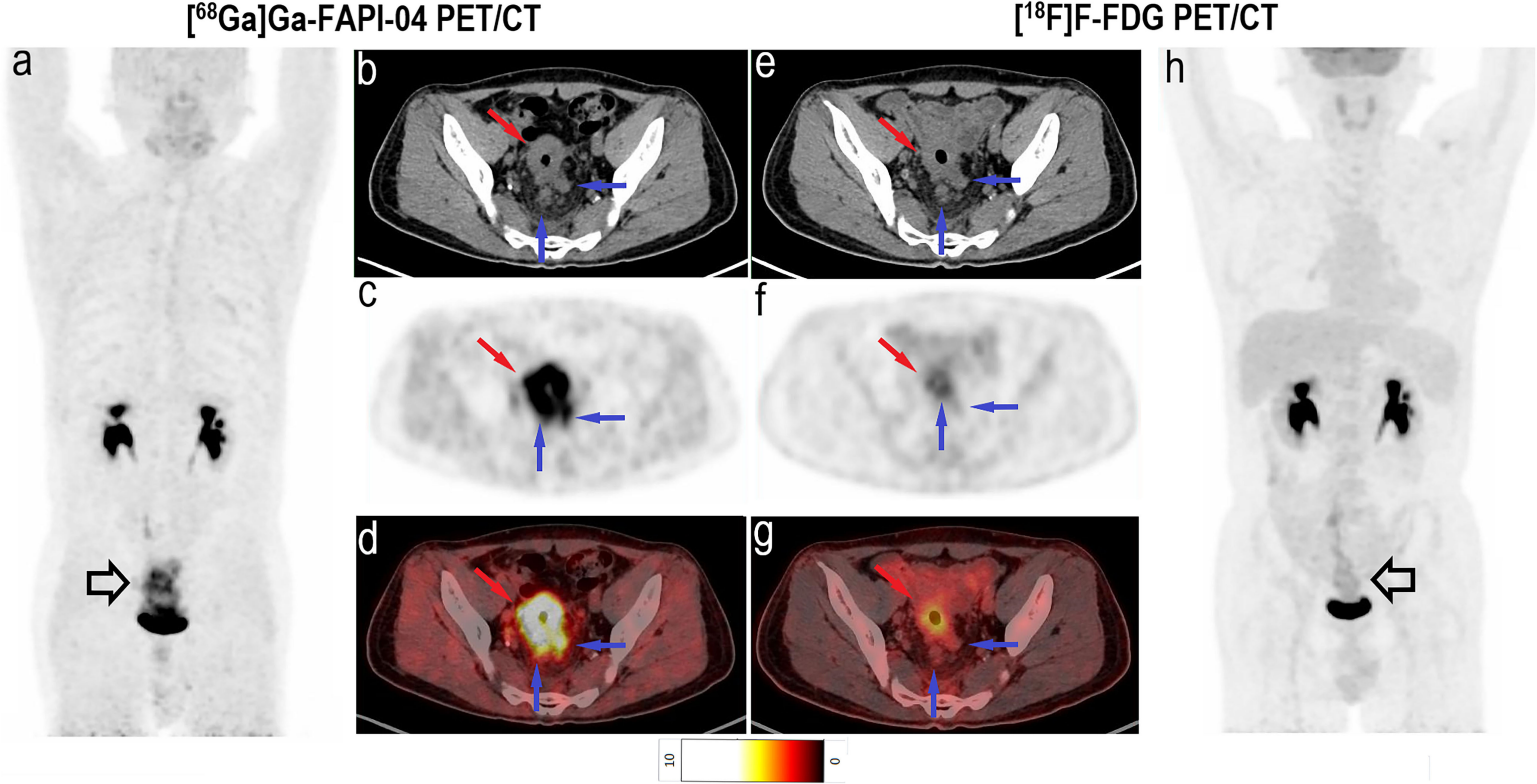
Images of a 49-year-old man with rectal mucinous carcinoma for staging. (A) Mean intensity projection images of [^68^Ga]Ga-FAPI-04 PET/CT scan. (B–D) Axial CT, PET and fused images of [^68^Ga]Ga-FAPI-04 PET/CT scan. (E–G) Axial CT, PET and fused images of [^18^F]F-FDG PET/CT scan. (H) Mean intensity projection images of [^18^F]F-FDG PET/CT scan. Compared with [^18^F]F-FDG PET/CT, [^68^Ga]Ga-FAPI-04 PET/CT exhibits the primary lesion (hollow black and red arrows) and suspicious lymph nodes (hollow black and blue arrows) more clearly because of the higher tracer uptake. The SUV_max_ of primary lesions was 14.6 for [^68^Ga]Ga-FAPI-04 and 4.4 for [^18^F]F-FDG PET/CT. The [^68^Ga]Ga-FAPI-04 PET/CT images also depict more intense tracer uptake in suspicious lymph nodes than [^18^F]F-FDG PET/CT images, with SUV_max_ values of 5.6–6.0 and 1.5–2.0, respectively.
